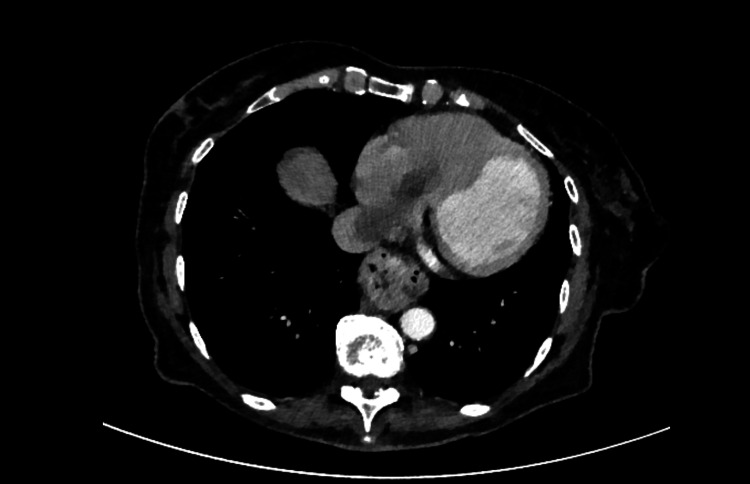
Axial CT chest with contrast CT chest which demonstrates the thrombus extending into the right atrium.
